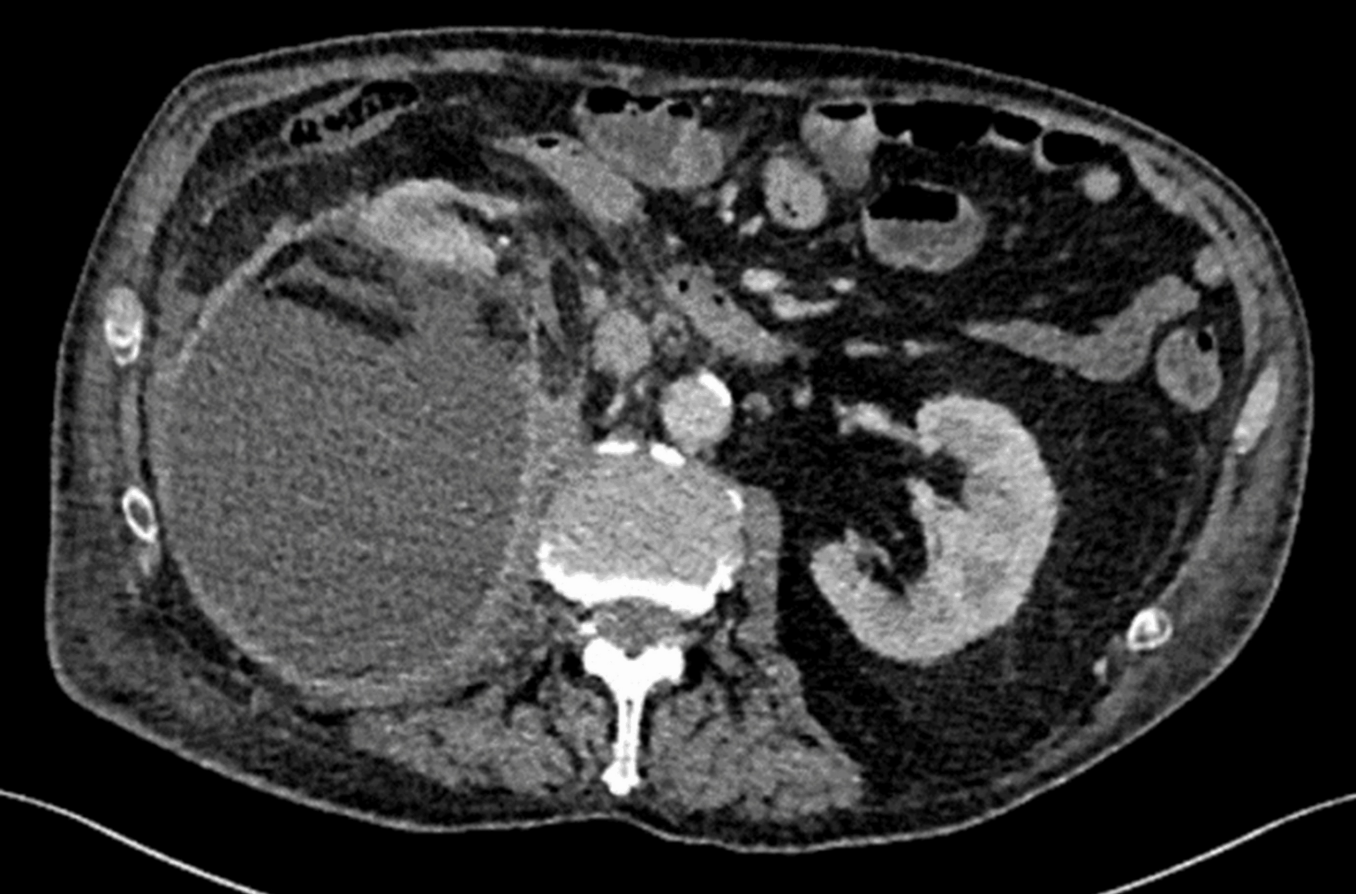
CT abdomen (axial view) in the portal venous phase CT demonstrates a significant increase in the size of the organised right perinephric collection, now measuring 24.5 × 13.0 × 11.4 cm, with concerns for superimposed infection. CT, computed tomography
